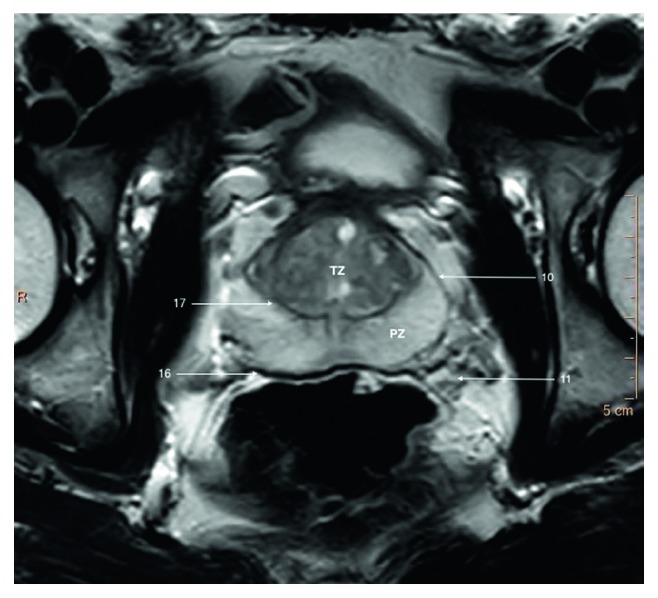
T2-weighted axial image of a prostate gland of a young patient showing clear zonal demarcation.
